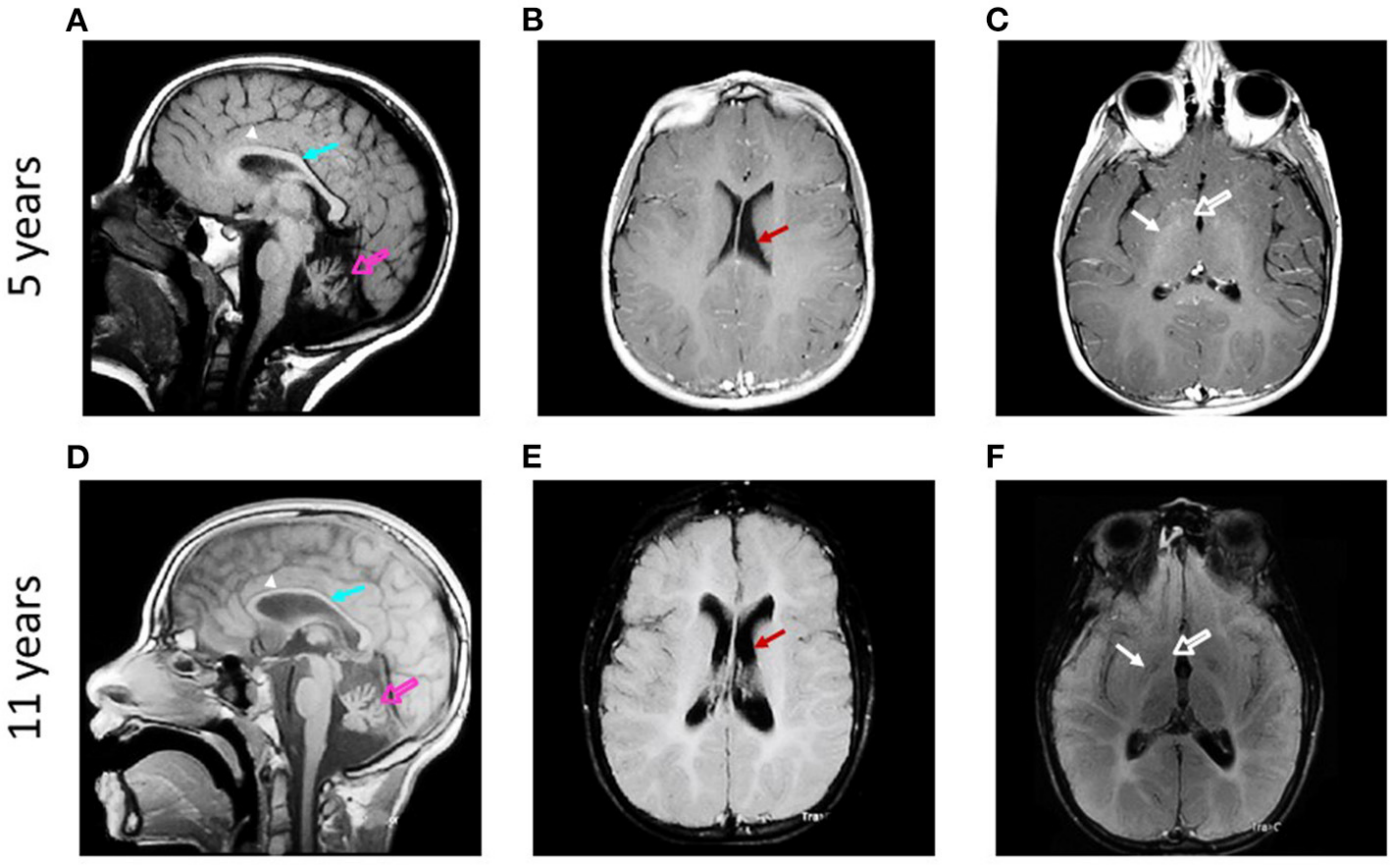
Qualitative analysis of the evolution of brain structures in a patient suffering from H-ABC. Sagittal T1-weighted magnetic resonance images obtained at 5 (A) and 11 years (D) show atrophy of cerebellum (open arrows) and corpus callosum (arrows) and involvement of U-fibers (arrowheads). Axial T2-FLAIR-weighted magnetic resonance images obtained at 5 (B,C) and 11 years old (E,F) show atrophy of the brain cortex and ventriculomegaly [arrows in (B,E)]. Atrophy of caudate (open arrows) and putamen (arrows) is also observed (C,F).
